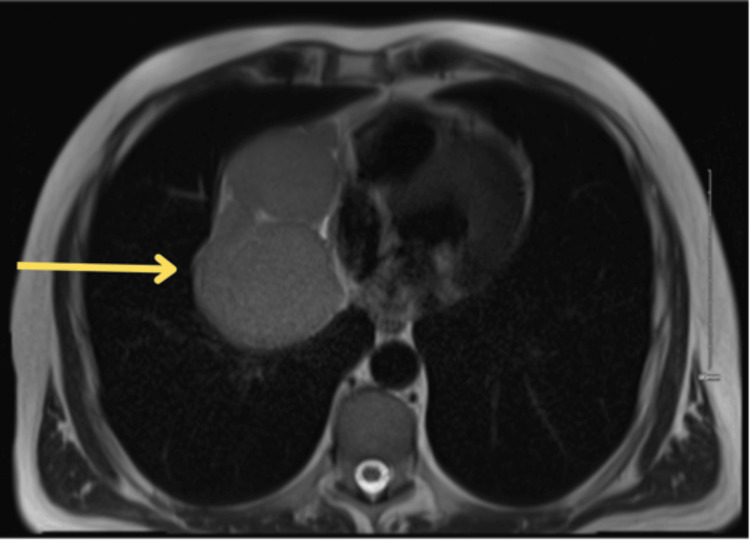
T2-weighted MRI chest pre-contrast phase showing a solid right-sided thoracic mass with internal septation (yellow arrow)
